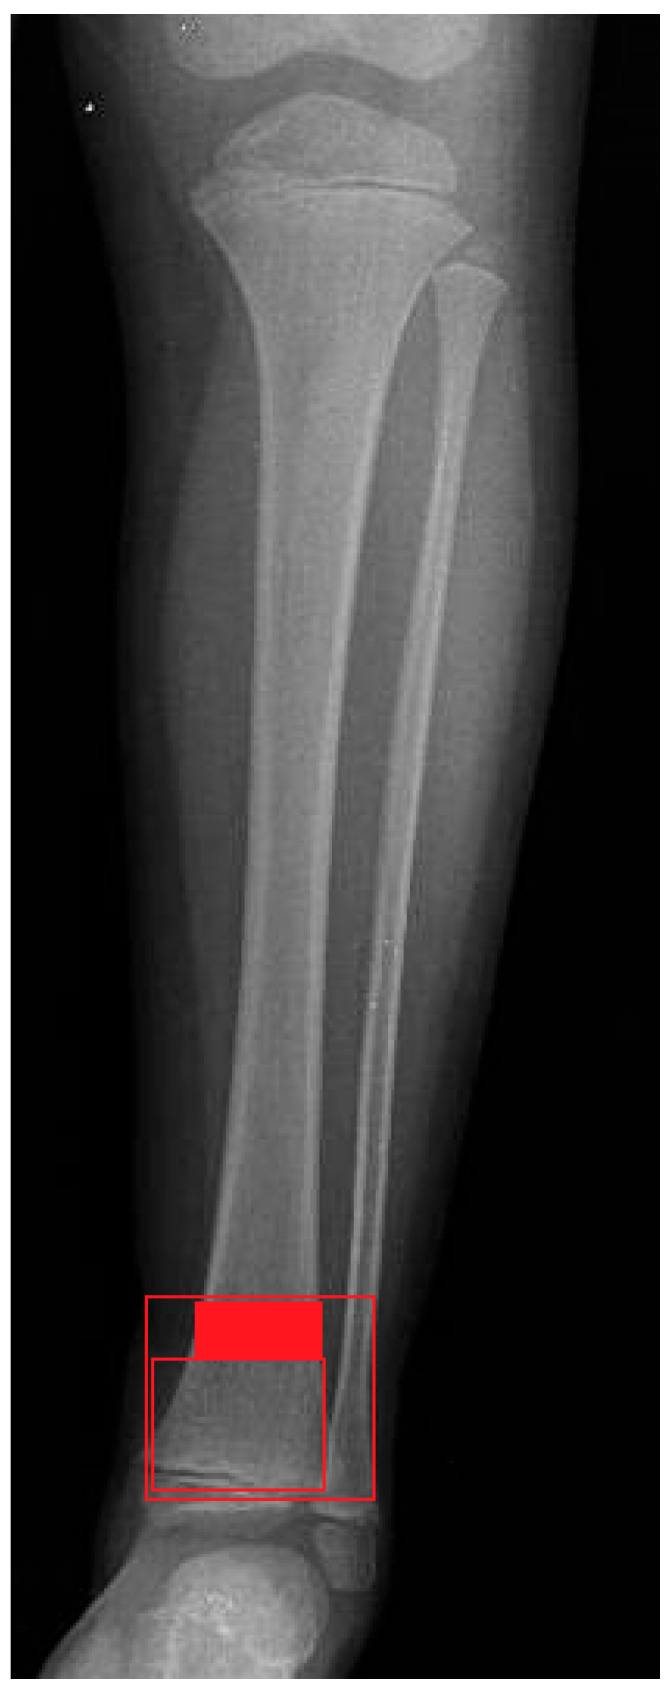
X-ray depiction of the DDMJ fracture.
